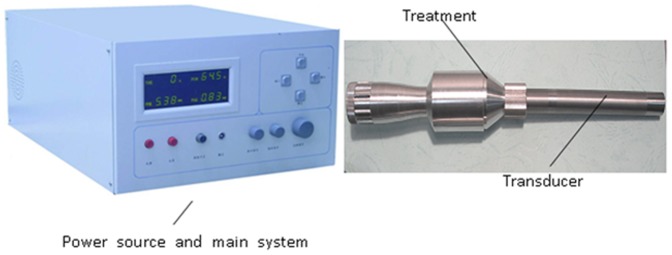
Focused ultrasound treatment system.
